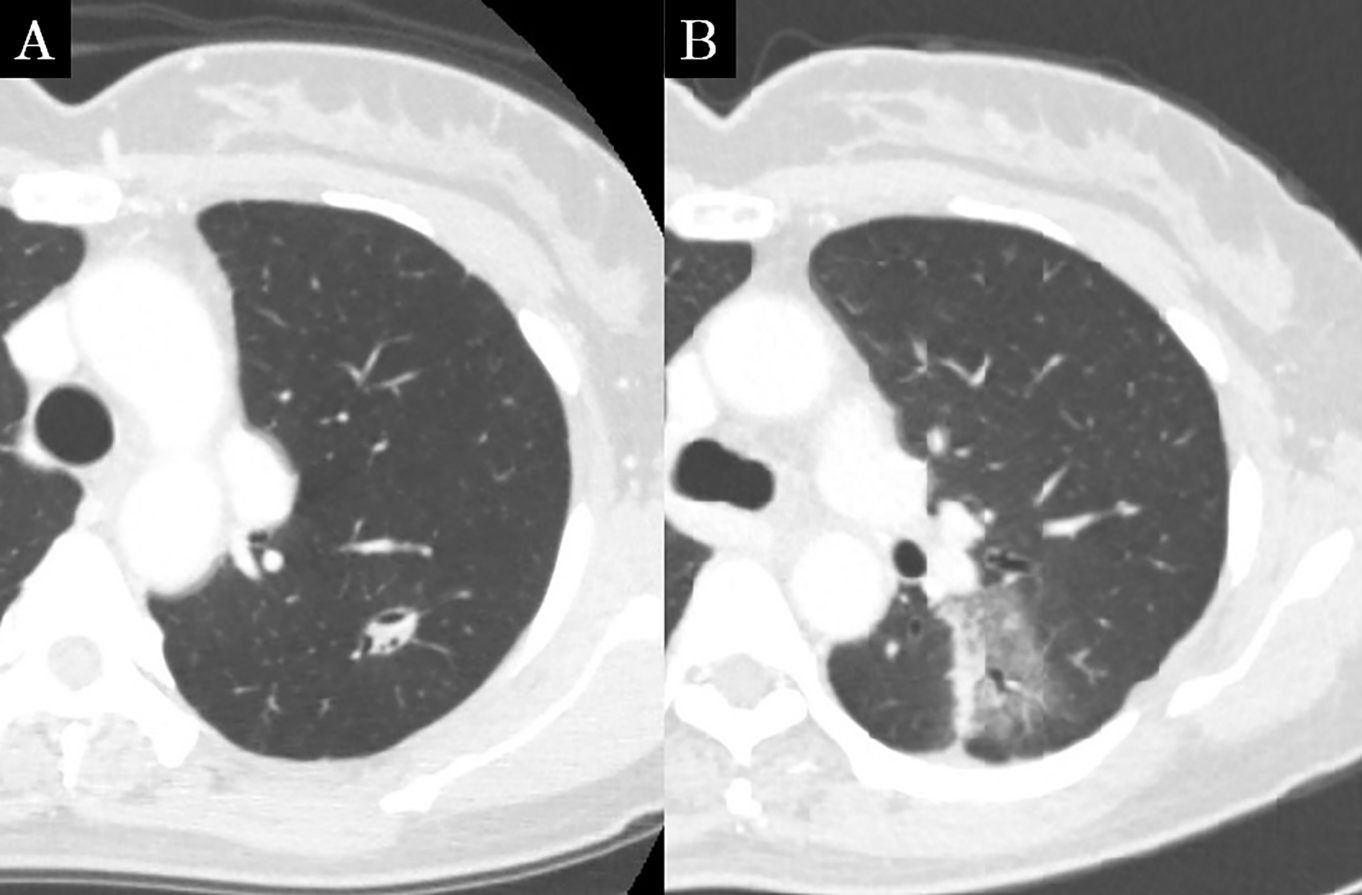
Concordance CT appearance, case 4. (a) Before SBRT, the lung tumor was located in the left lower lobe. (b) Patchy areas of hazy are seen; this shadow was diagnosed as patchy GGO at 3 months after SBRT.
